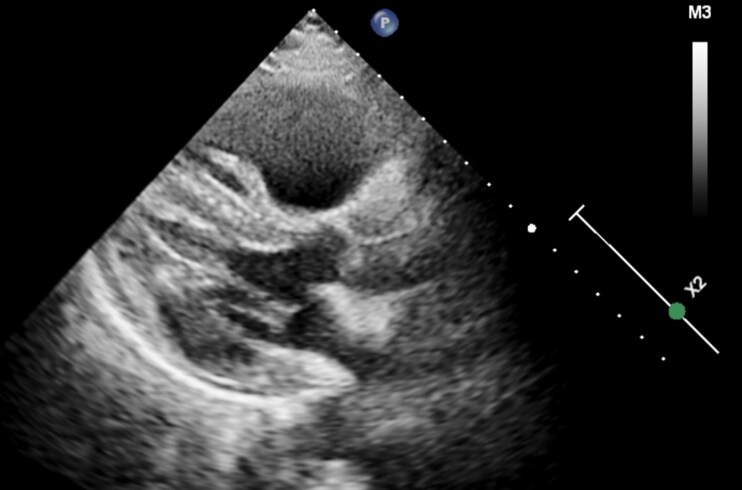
Echocardiography in the parasternal long-axis view, showing an enlarged right ventricle and an underfilled left ventricle.
